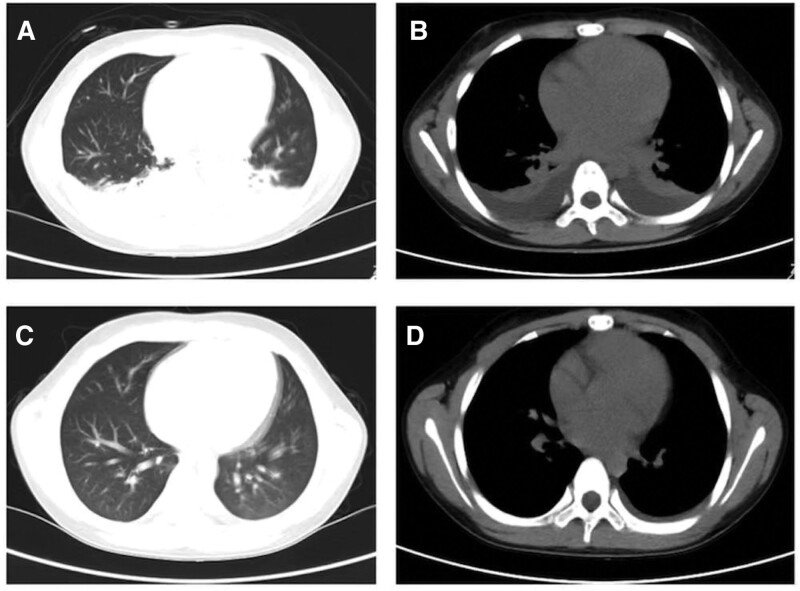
Lung consolidation and pleural effusion on CT. (A, B) Chest CT during the acute phase of disease revealed lung consolidation and pleural effusion. (C, D) Lung consolidation and pleural effusion gradually absorbed after treatment. CT = computerized tomography.
